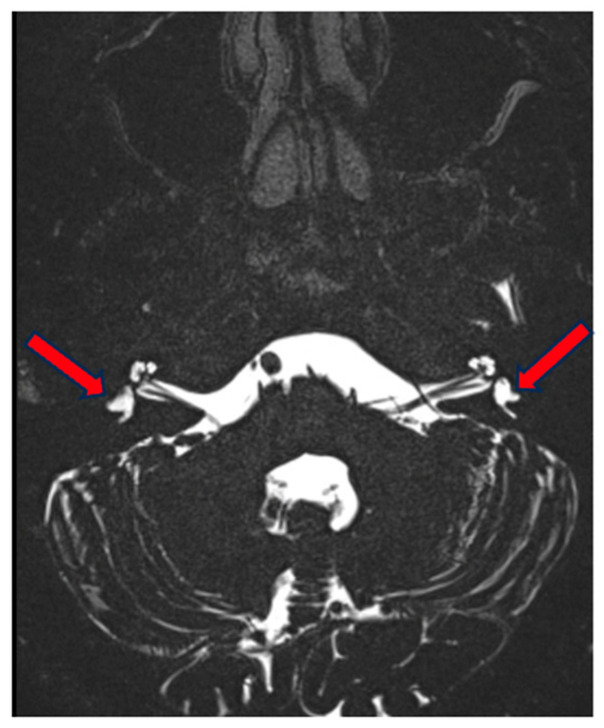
MRI of the inner ear for case 3, T2 tse3d weighted image, hypo-/dysplastic lateral semicircular canals bilaterally (red arrows).
